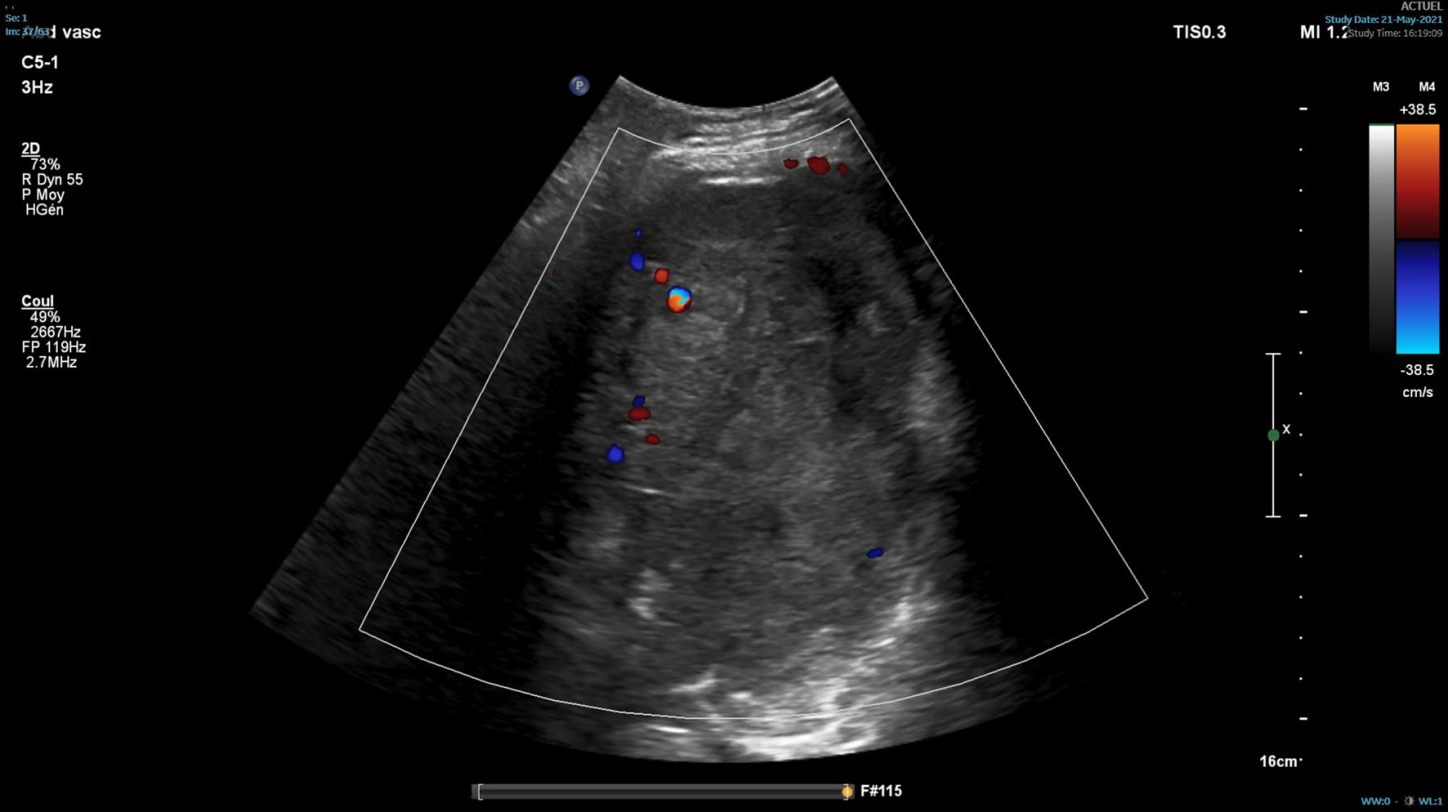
Doppler ultrasound image of the right scapular mass after thirteen sessions of lesion-focused radiotherapy showing less intra-metastatic arteriovenous shunts
